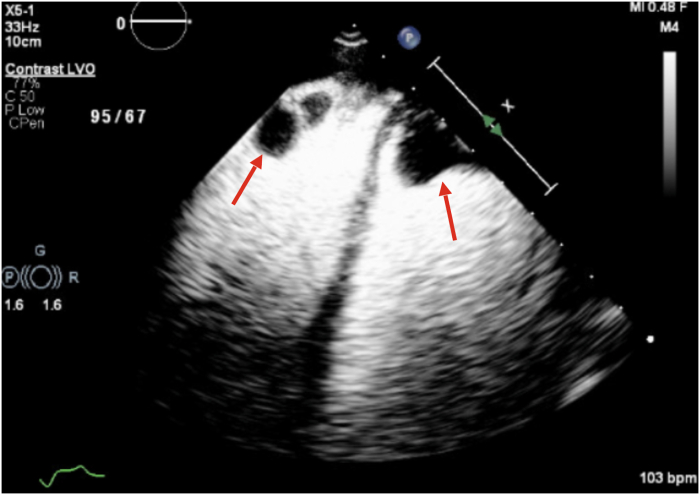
Initial Transthoracic Echocardiogram With Contrast Demonstrating Right and Left Ventricular Thrombi (Arrows)
